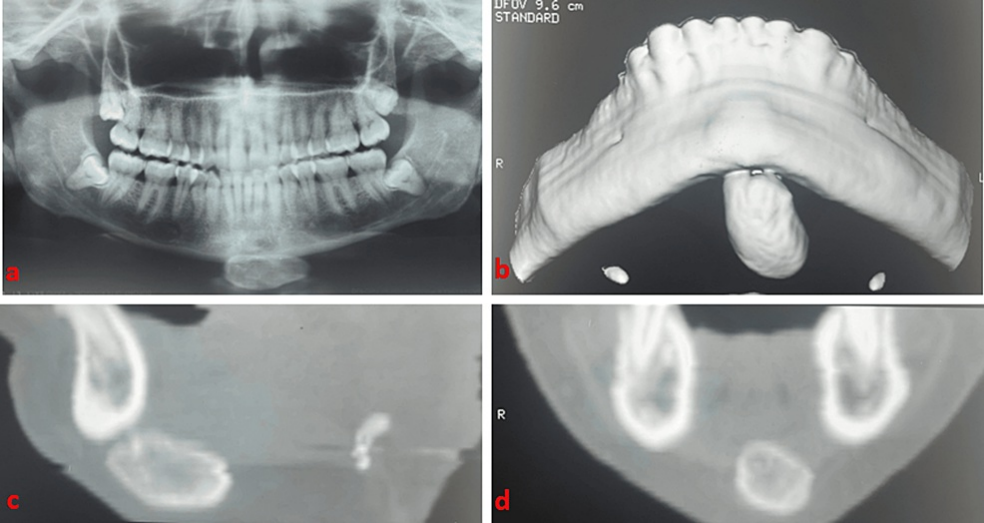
(a) Panoramic radiograph; (b) 3D CT image; (c) sagittal CT image; and (d) coronal CT image.
